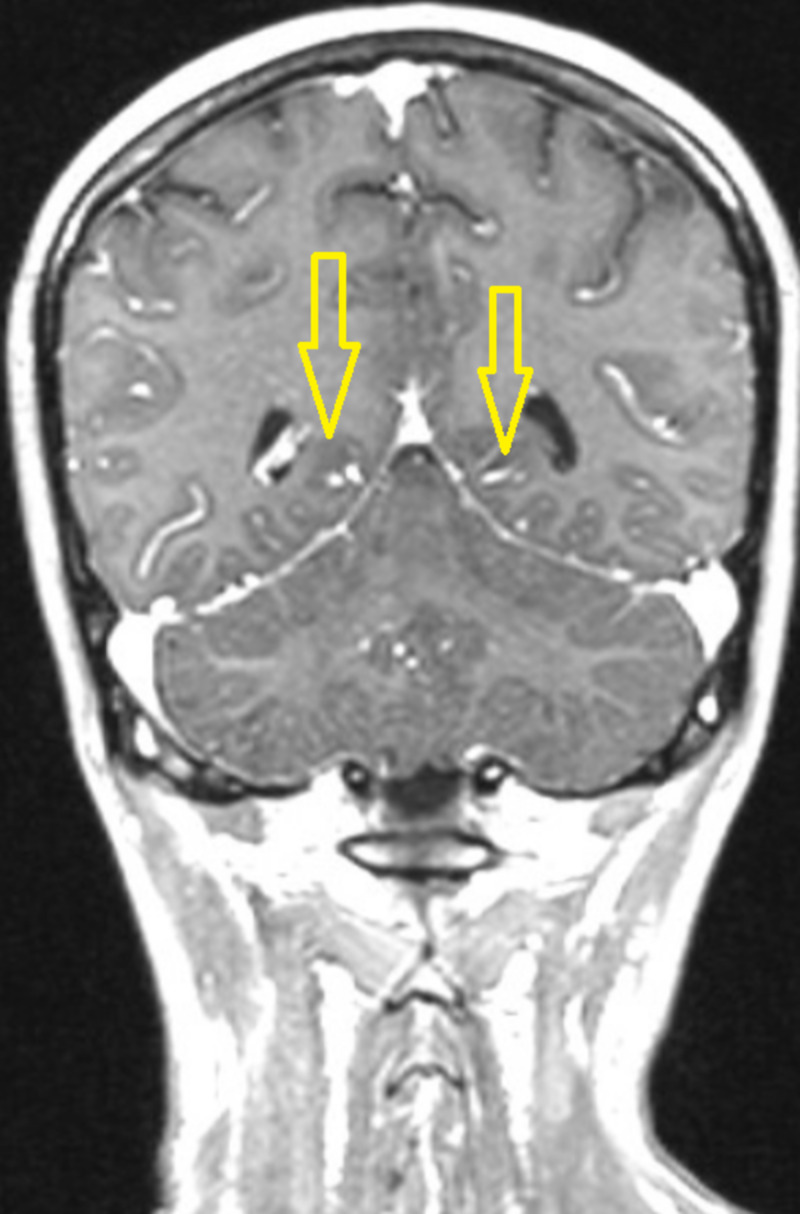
The coronal post-contrast T1 weighted brain MRI image demonstrates leptomeningeal enhancement (yellow arrows).
